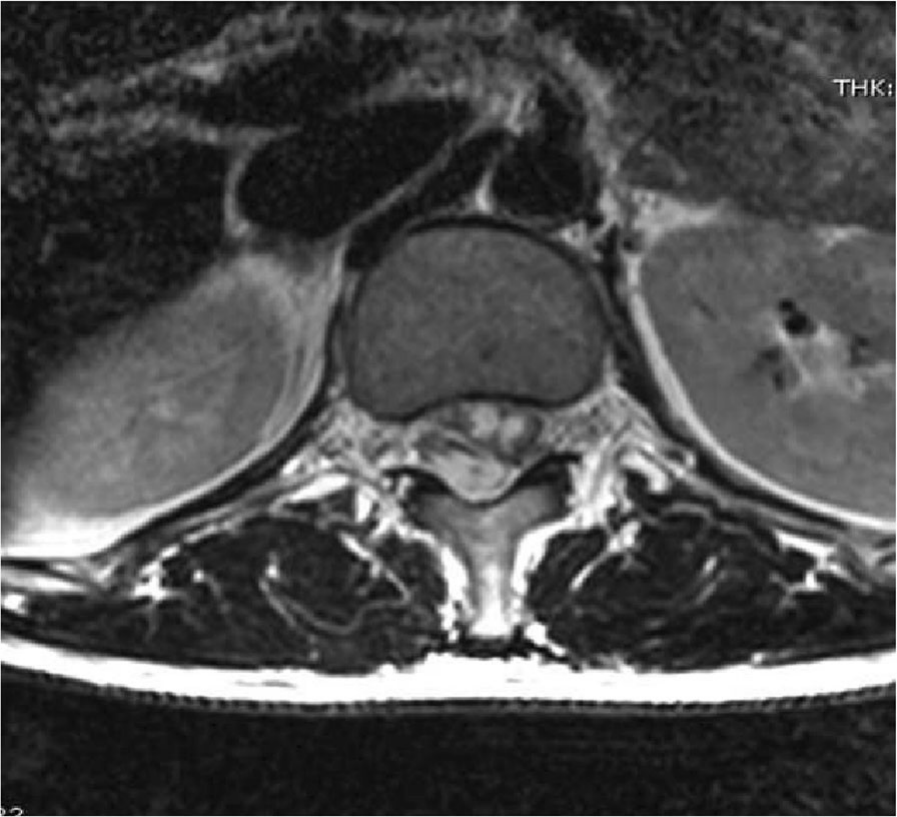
Axial T2-weighted MRI shows hematoma compressing spinal cord from the right side
